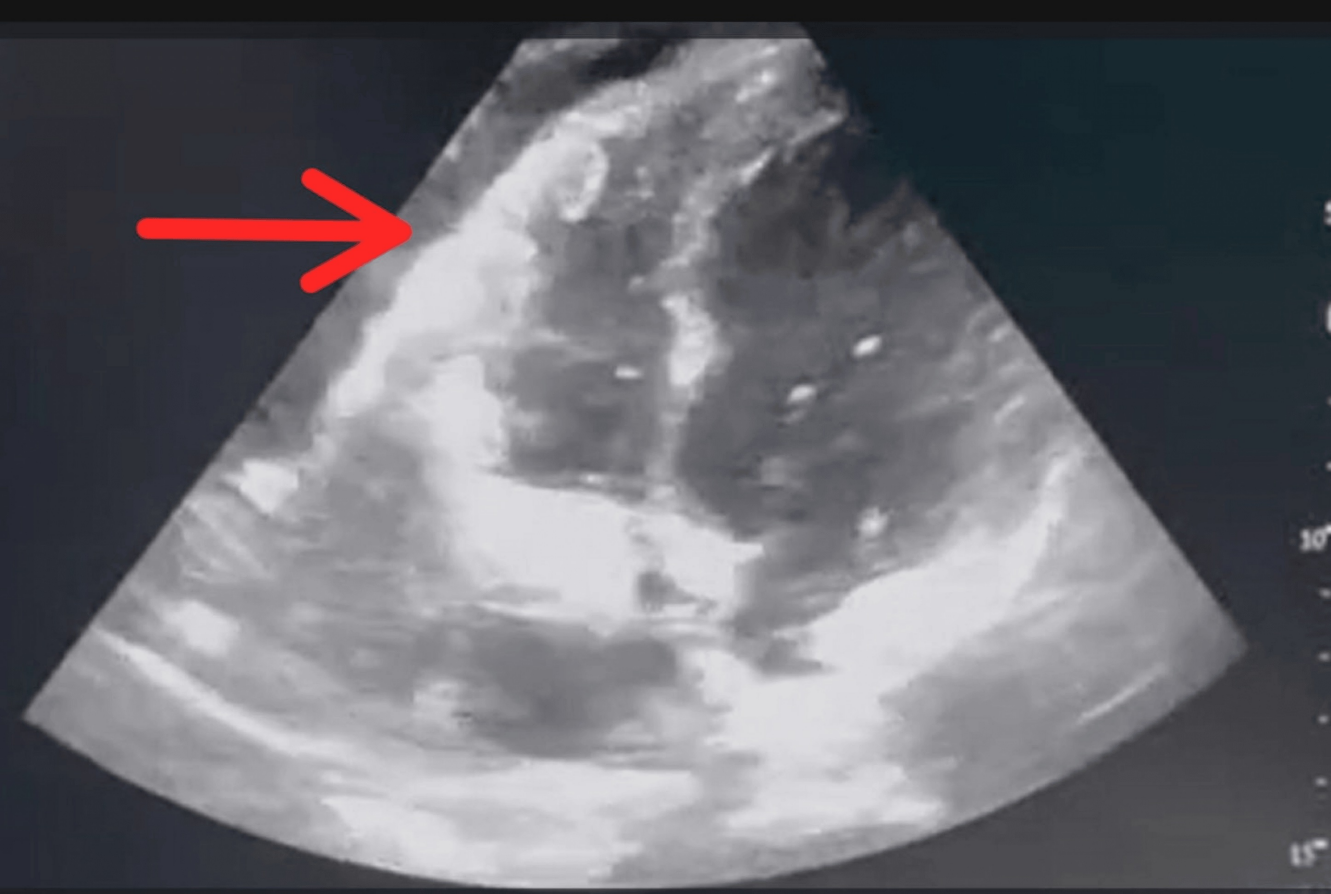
In apical four-chamber view on 2D echocardiography, moderate pericardial effusion can be seen (red arrow)
